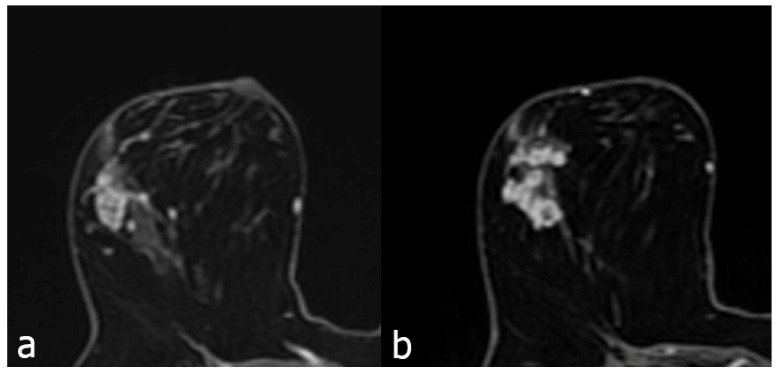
Contrast enhanced breast MRI, focal heterogeneous enhancing NME lesion (a), biopsy result of DCIS in a 45-year-old patient. Second image (b), 52 years old, focal clustered-ring enhancing NME lesion, invasive carcinoma as a result of biopsy.
